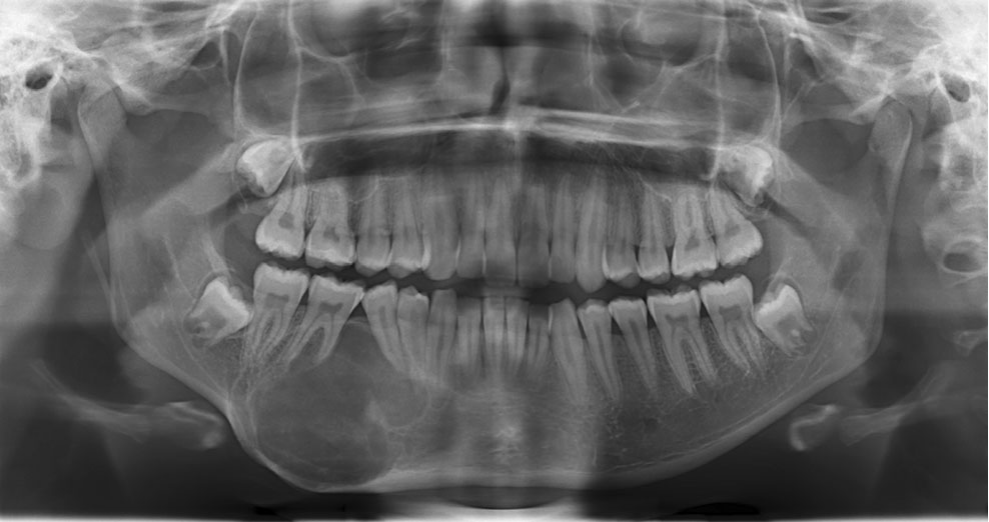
An orthopantomogram of an 18-year-old female that demonstrates an ossifying fibroma of the right body of the mandible. The solitary expansile lesion splays the teeth and has a discernible sclerotic rim, but no destructive features, e.g., root resorption
